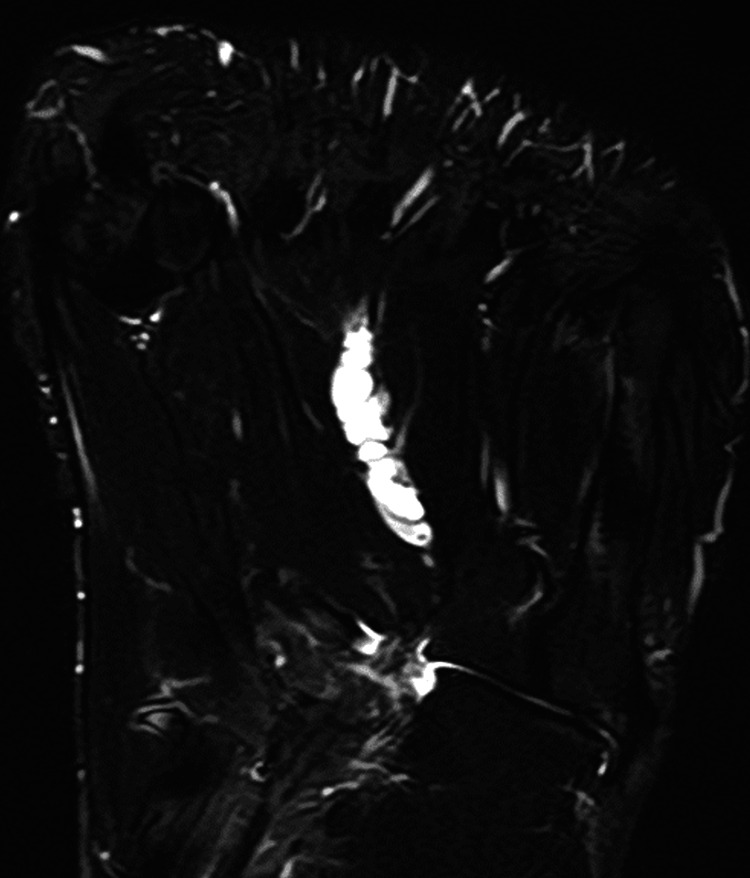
MRI of the right foot on the plantar aspect showing bead-like cysts.
